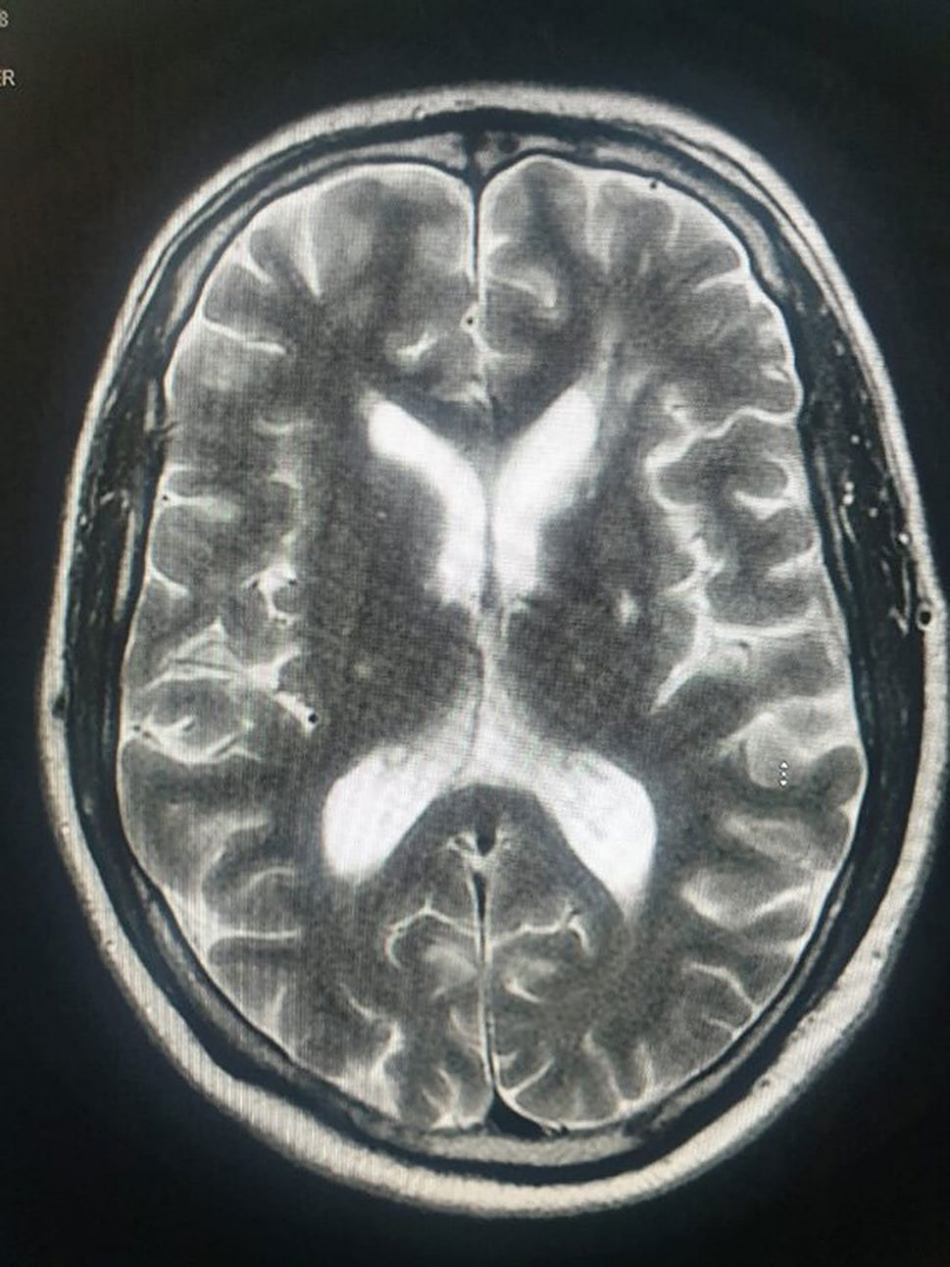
Magnetic resonance imaging utilizing stroke protocol showing small foci of high T2/FLAIR signal intenisty
